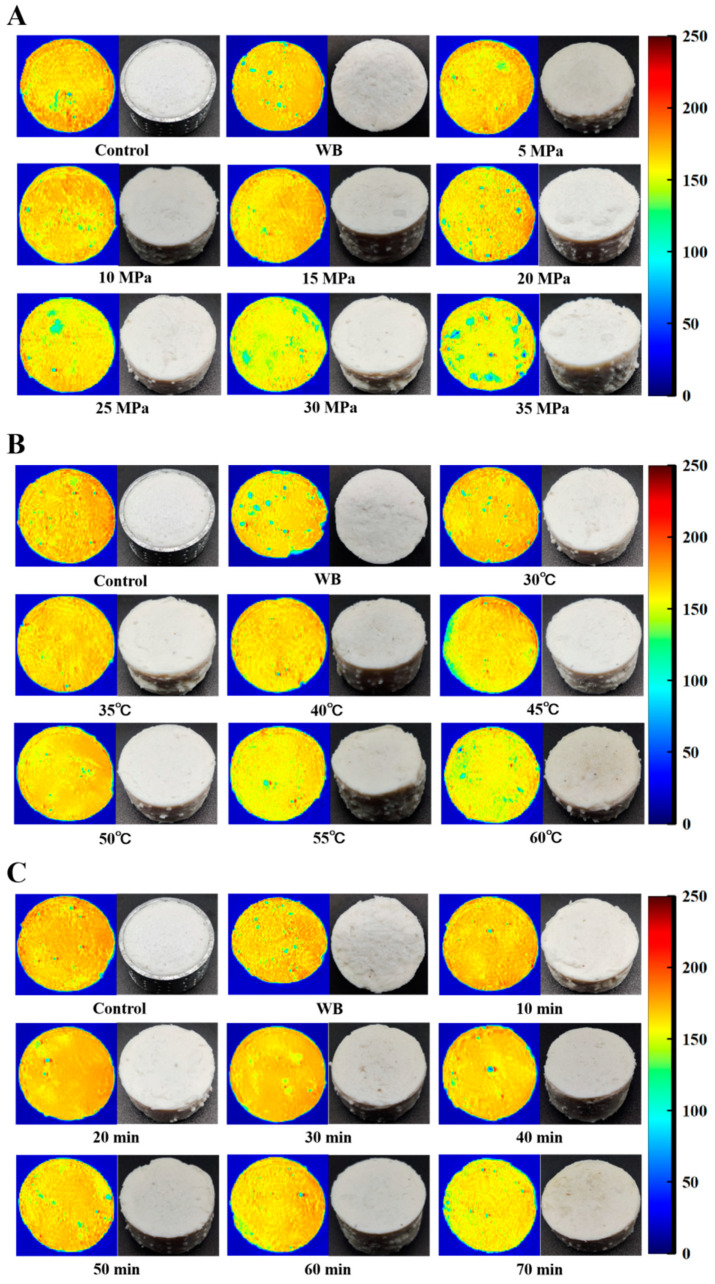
Effect of different DPCD treatments on MRI of surimi (A): Pressure, (B): Temperature, (C): Time.
